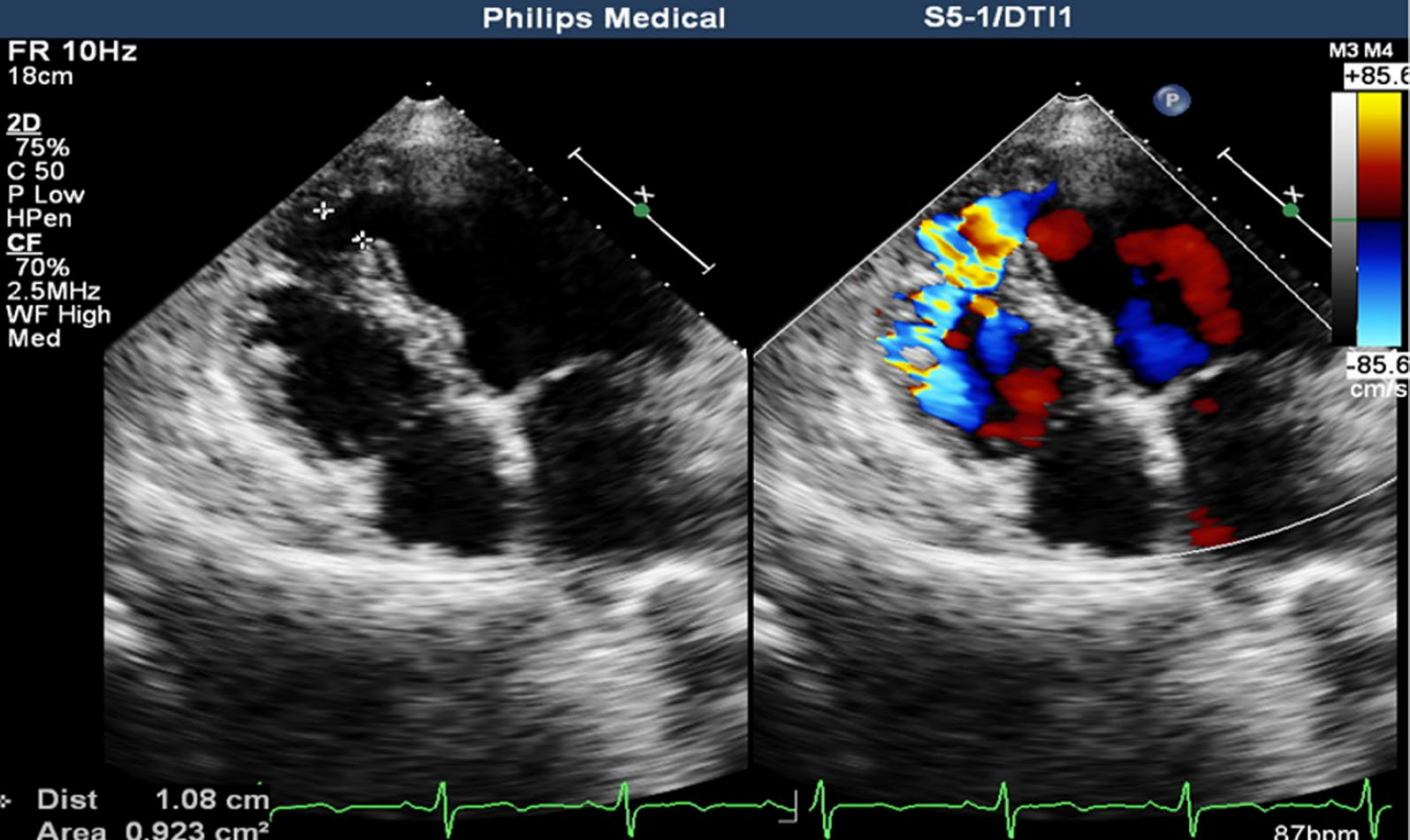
The patient’s cardiac ultrasound results after admission (apical ventricular septal had a defect of 10.8 × 4.1 mm; left-to-right shunt of colored blood flow)
